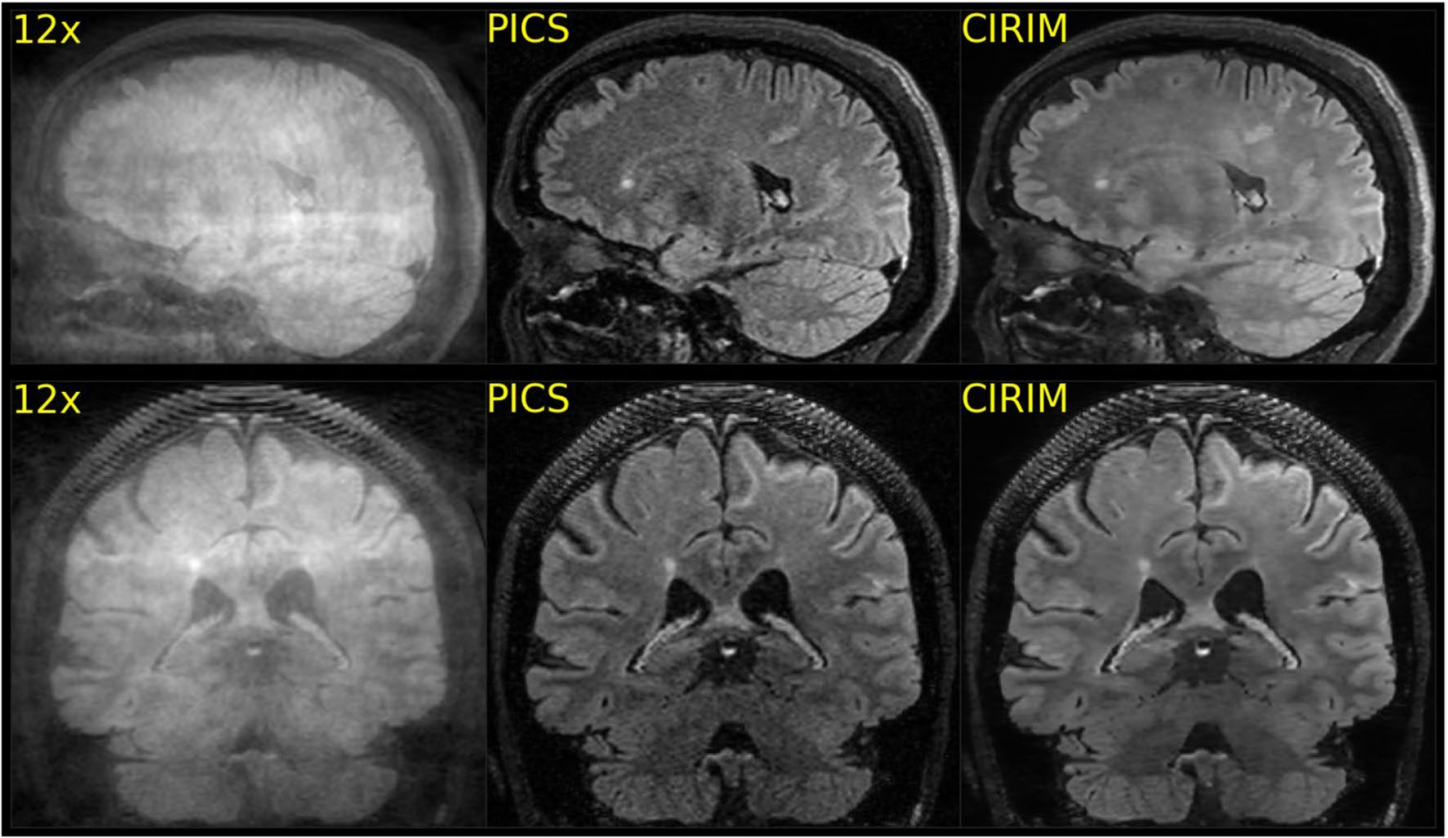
Reconstructions of 12 times accelerated FLAIR scans of two subjects, where the CIRIM and PICS provided high-quality reconstructions but were interpreted differently by two raters in a side-by-side comparison. Rater 2 interpreted the CIRIM reconstructions as having sharper edges, whereas Rater 3 interpreted the grainier PICS reconstructions as resulting in increased sharpness
